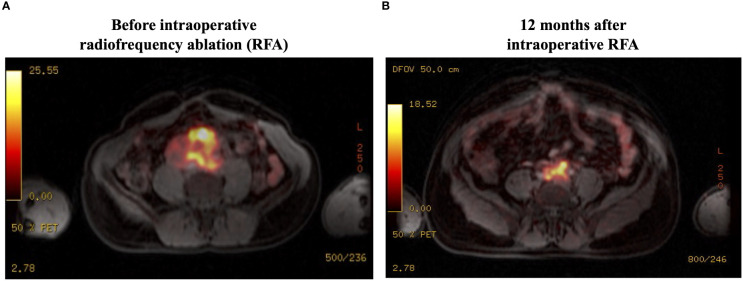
Ga^68^-DOTATATE PET-MRI findings: (A) There is high uptake (SUVmax, 32.5) in the abdominal mass, with local invasion but without distant metastatic disease. (B) There is a significant reduction in the tumor uptake (SUVmax, 18.5) at 12 months after RFA. SUVmax, maximum standardized uptake value; RFA, radiofrequency ablation.
